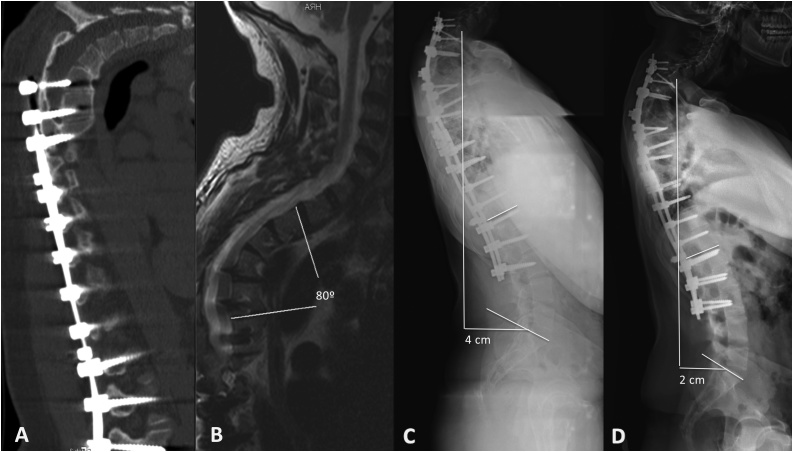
A: Sagittal images of the computed tomography (CT) study, with proximal kyphosis of 80° T2–T5 without correction in decubitus position. B: Sagittal images of the magnetic resonance (MR) study, in which no spinal changes are observed in the apical thoracic region. C: Postoperative radiologic study after performance of pedicle subtraction osteotomy at the level of the apical region of the deformity (T3), with proximal extension of the instrumentation showing adequate correction of 30° with residual deformity of 50°. Negative sagittal balance (sagittal vertebral axis) (SVA) of 4 cm. D: Twelve months after the intervention the patient referred pain and cervicothoracic area showed deformity and swelling. In radiological study, pulling out at C7 level and T1–T4 kyphosis of 53° were observed, but findings suggested fused PSO level. Radiologic study showed pulling out at C7 level and low increase of T1–T4 kyphosis (53°) and negative sagittal balance (sagittal vertebral axis) (SVA) of 2 cm.
